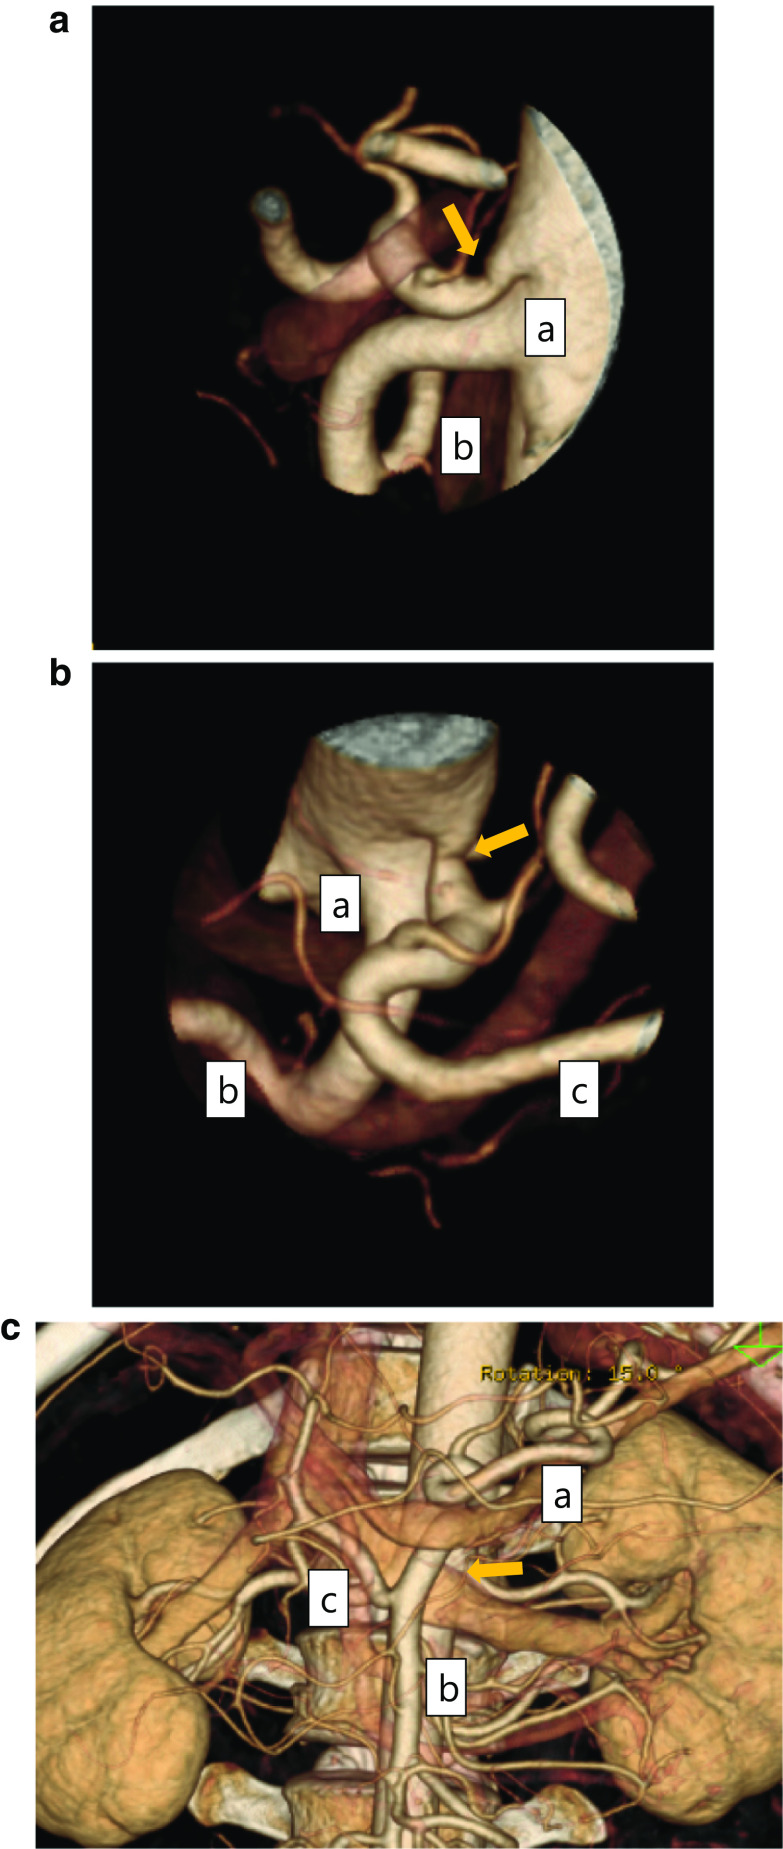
Preoperative abdominal computed tomography scan. a, b The origin of the celiac artery was stenosed due to compression of the median arcuate ligament (MAL): a superior mesenteric artery, b common hepatic artery, c splenic artery. c Adachi V vascular anomaly was detected: a splenic artery, b superior mesenteric artery, c common hepatic artery
